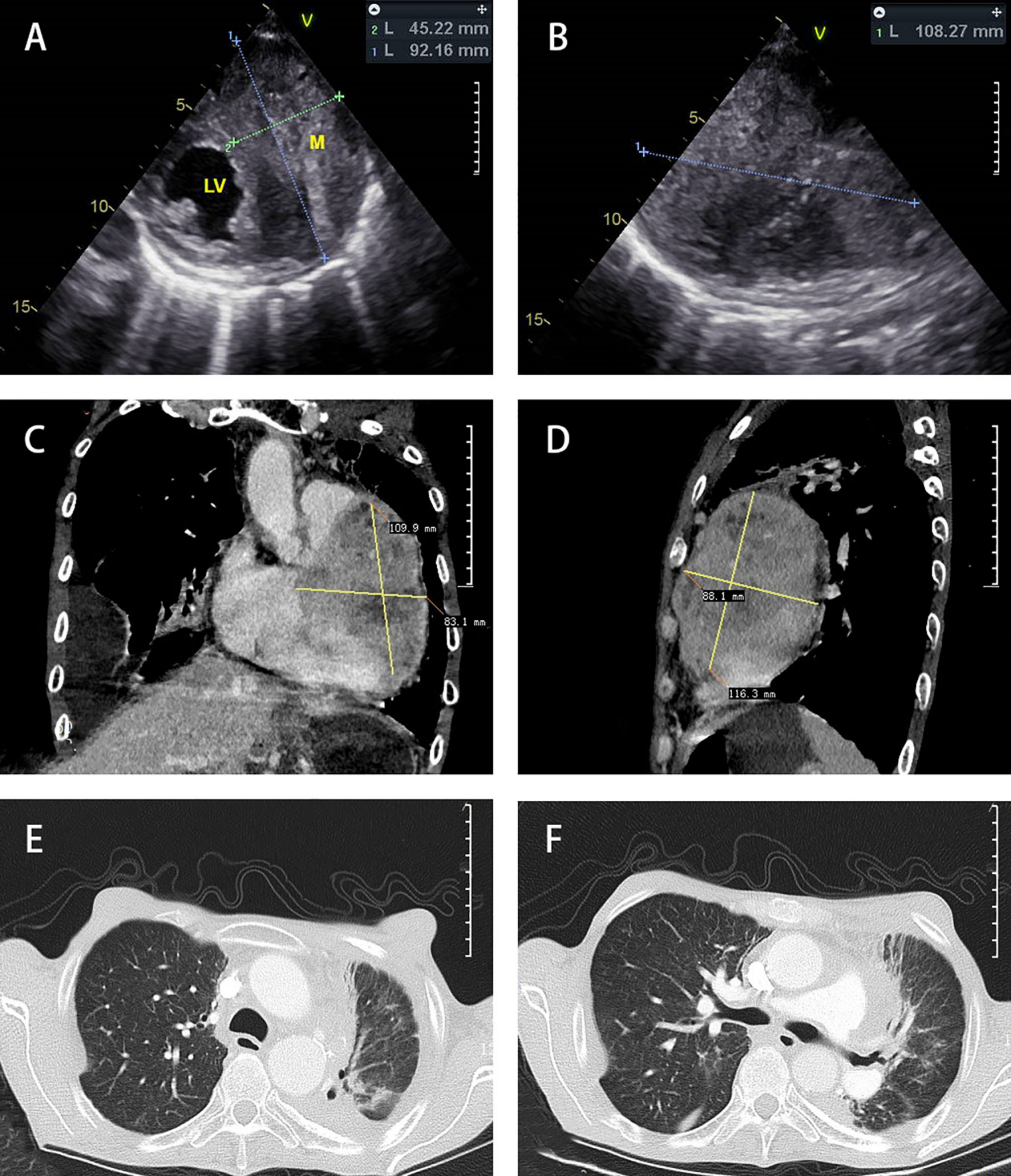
Diagnostic imaging. Echocardiography (A, B) showed a hypoechoic mass in the left ventricle and the anterolateral right ventricle. Chest CT scan mediastinal window (C, D) with contrast enhancement showed that the heart shadow had increased. A round, soft-tissue mass was observed at the left edge of the Cardiac margin. Multiple lymph node shadows were observed at the left hilum of the lung and mediastinum. The chest CT scan lung window (E, F) showed right pleural effusion and bilateral pneumonia.
